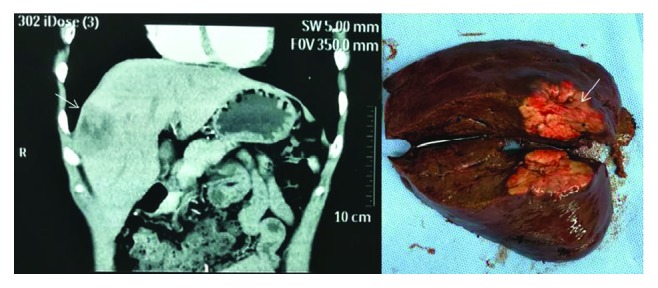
Findings of computed tomography: sagittal plane of computed tomography shows the low-density area (arrowhead) at the right anterior lobe of the liver. Macroscopic findings: gross appearance of the resected liver shows a gray-white tumor measuring 34 mm × 32 mm × 19 mm (arrowhead).
